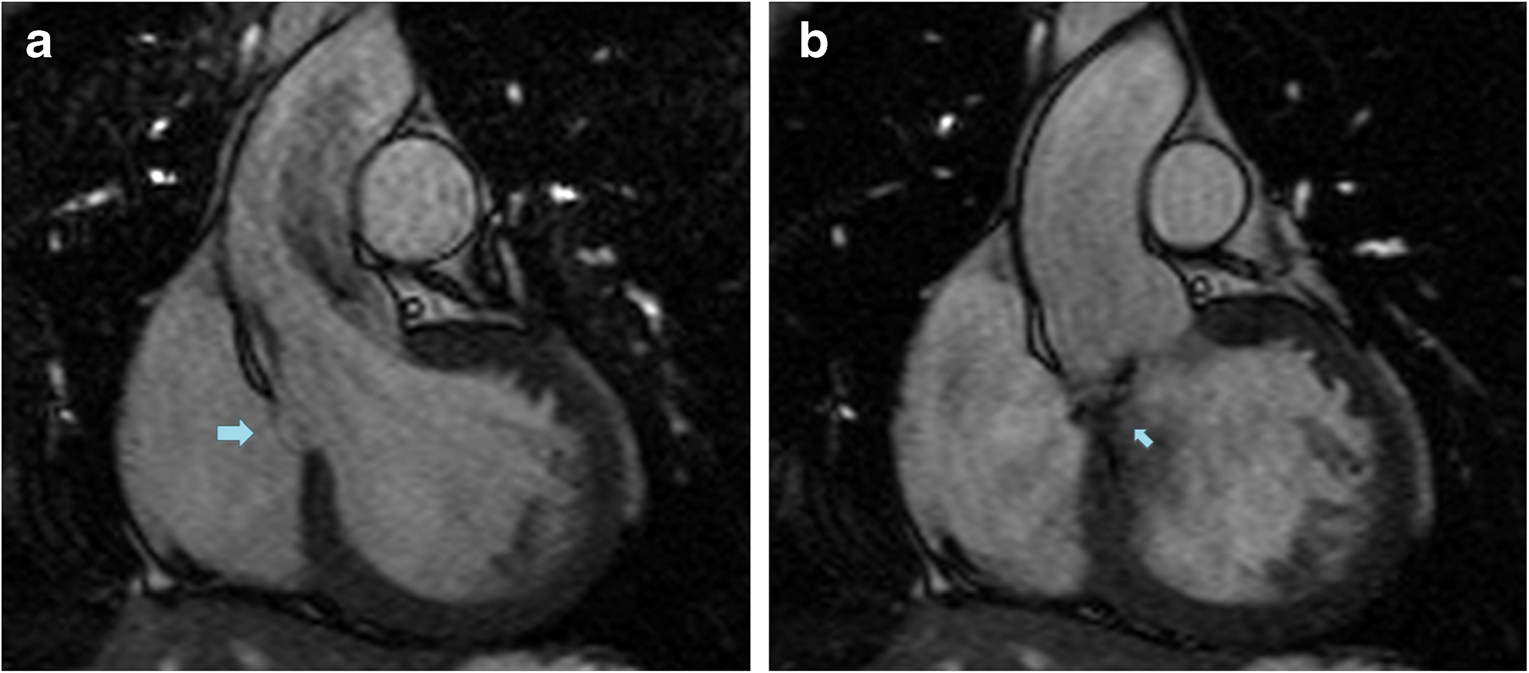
33-year-old man with bicuspid aortic valve and aortic insufficiency. Coronal oblique MRI (bSSFP sequences) through the LVOT shows a small aneursymal out-pouching of the IVMS (*arrow*). Systolic image (a) shows dephasing artifact associated with a post-stenotic jet from aortic stenosis in a bicuspid valve, while a diastolic image (b) shows an eccentric regurgitant jet (*small arrow*) directed towards the IVMS aneurysm
